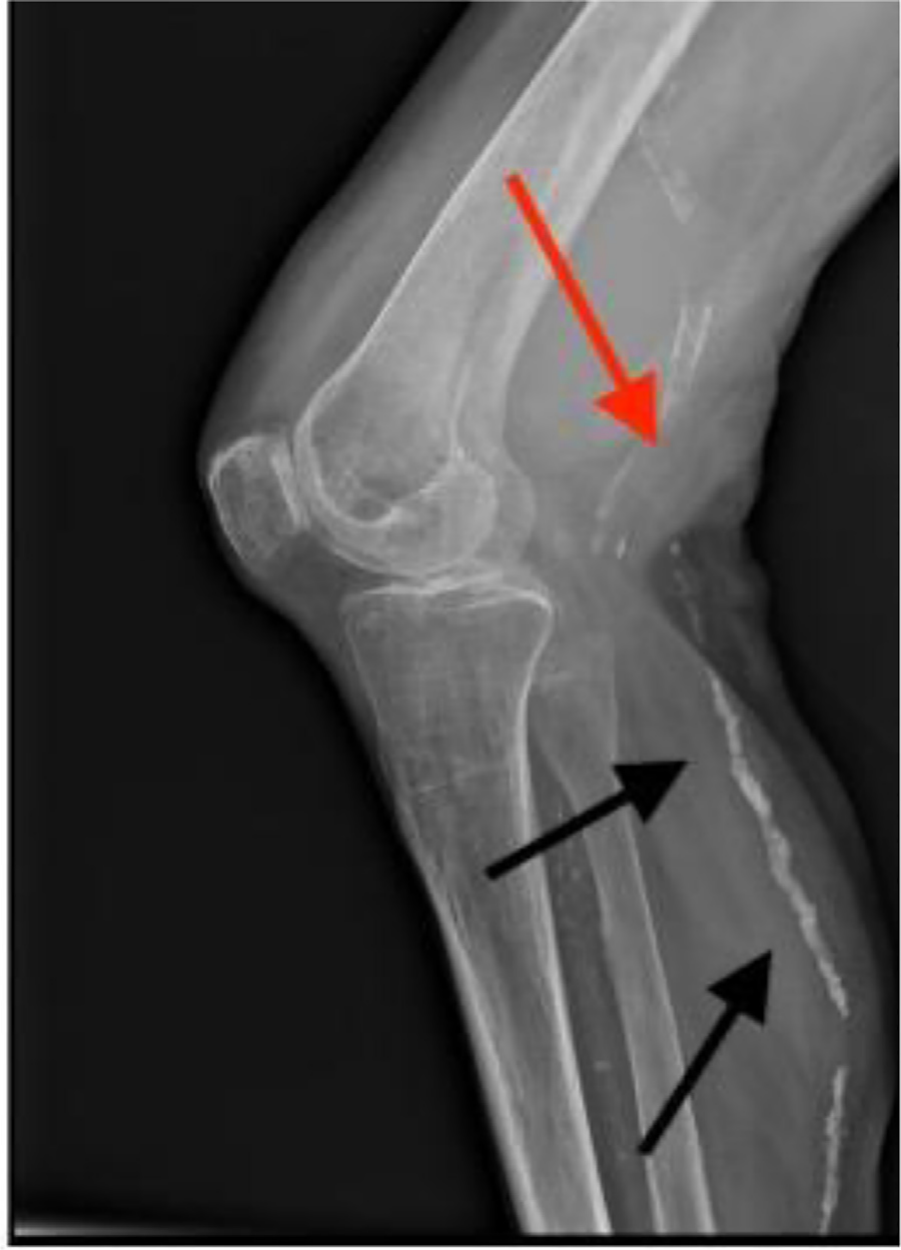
X-ray of the left knee joint, lateral view. Legend 1: Backward arched displacement of the calcified popliteal artery, black arrows indicate the calcified vein and red arrow marks the expansion already visible in the x-ray caused by the pseudoaneurysm of the popliteal artery.
